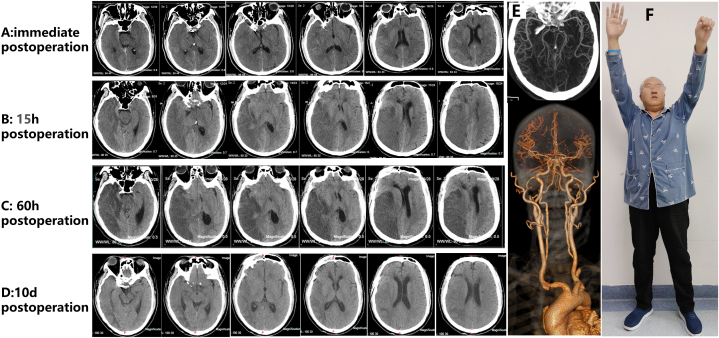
Postoperative follow-up. A: The head CT scan - immediate postoperation showed large hypodense foci in the right cerebral hemisphere with a midline shift. B. The head CT scan - 15 hours postoperation showed large low-density foci on the right side, and the brain tissue was swollen. C. The head CT scan - 60 hours postoperation showed large low-density foci on the right side. D. The head CT scan −10d postoperation showed the centered midline. E. Head and neck CTA -10d postoperation showed the siphon segment of the right ICA was stenotic (70 %). F. The patient's mRS score was grade 2 at the 2-year follow-up.
